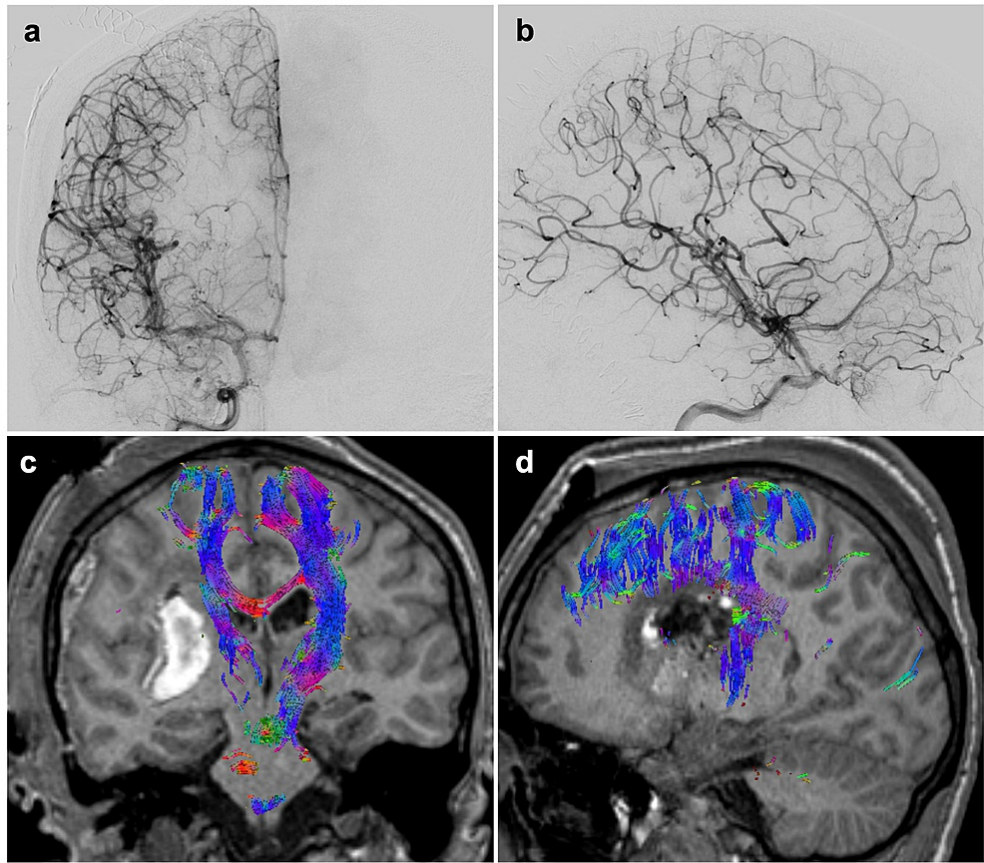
Post-operative MRI with DTI and angiogram. (A, B) Post-operative MRI with DTI sequence in the coronal (A) and sagittal planes (B) showing preservation of the CST. (C, D) Post-operative lateral and AP angiography displaying complete resection of the AVM nidus. DTI, diffusion tensor imaging; CST, corticospinal tract; AVM, arteriovenous malformation; AP, anteroposterior.
